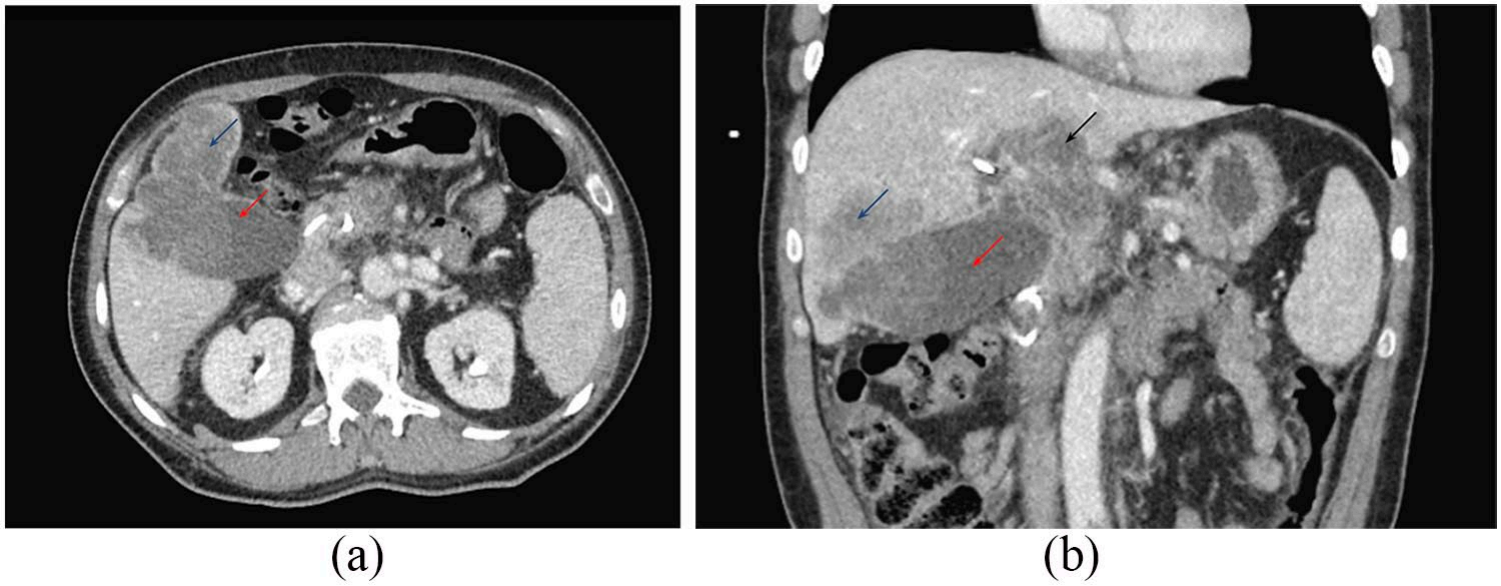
(a) CT scan at date of admission. Gallbladder lesion within the fundus
